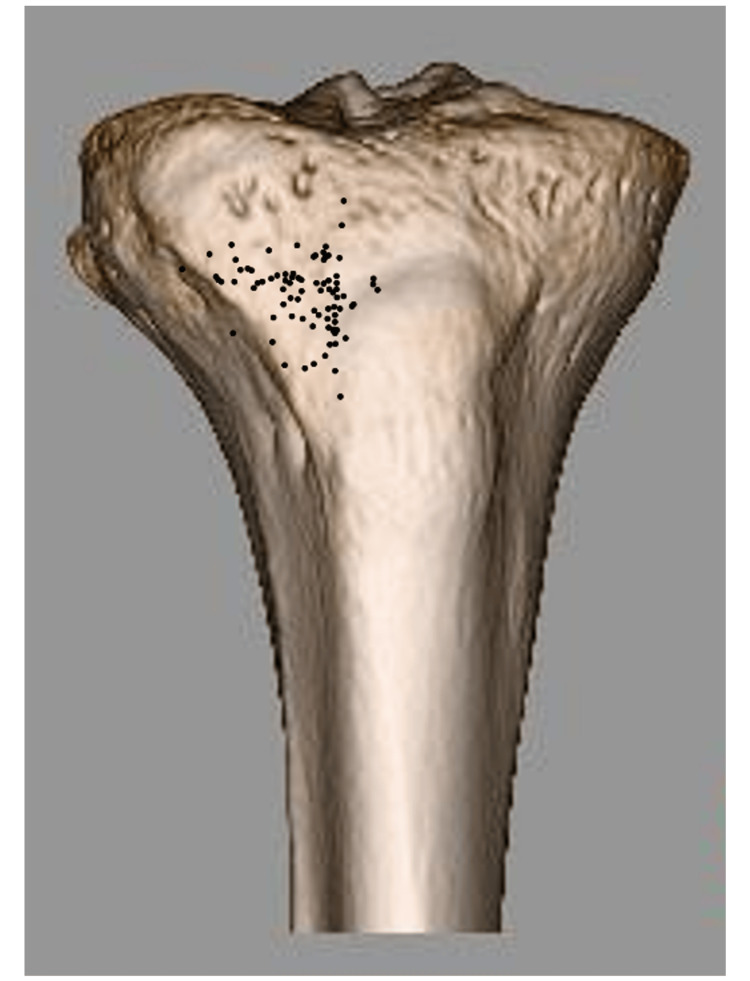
Distribution of anatomical vector destinations of the medial meniscus posterior root (MMPR) Each black dot represents the distal destination point of the anatomical vector of the medial meniscus posterior root on the tibia, based on MRI analysis.
